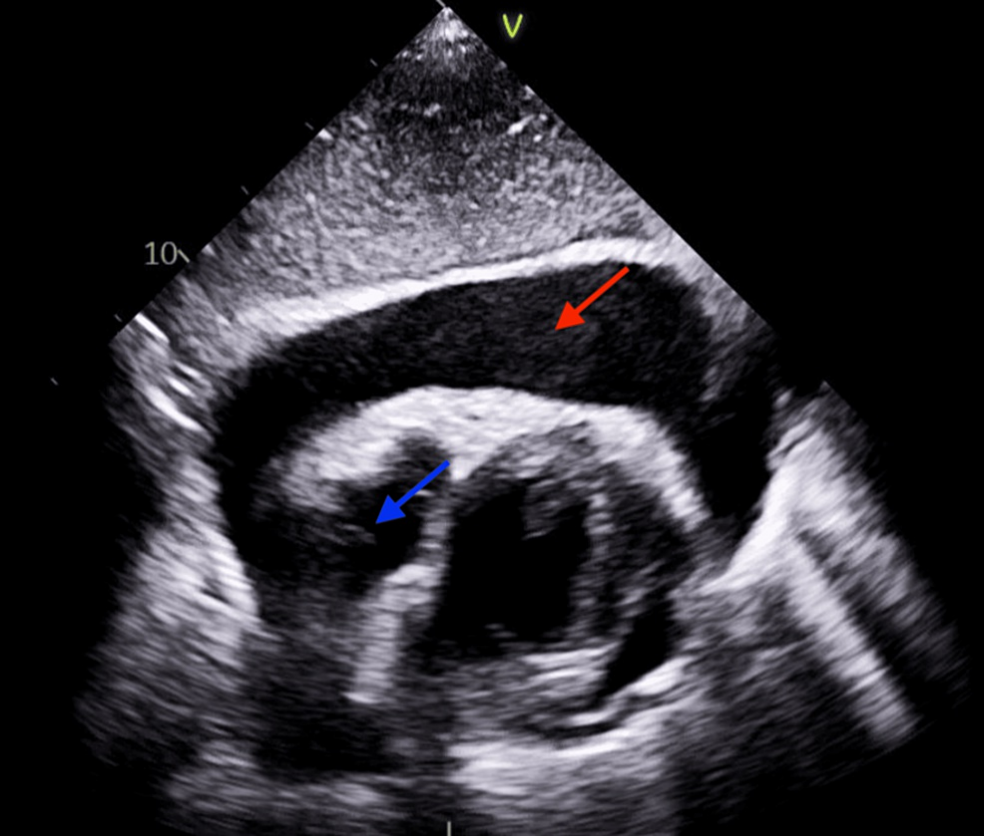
Transthoracic echocardiogram (TTE) showing pericardial effusion TTE showing large pericardial effusion (red arrow) and collapsed right atrium (blue arrow)
